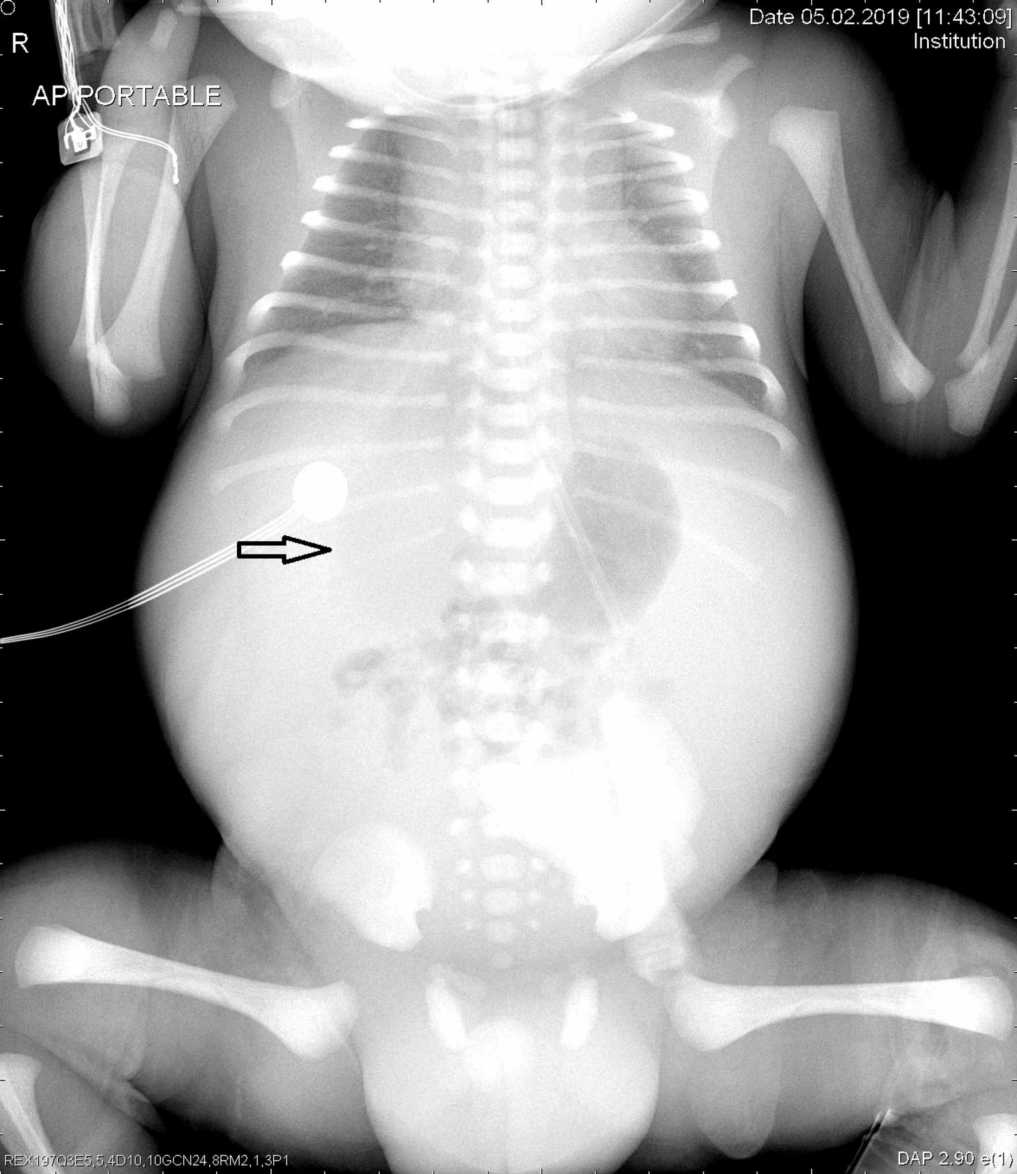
X-ray of abdomen supine. Arrow showing circular faint lucency in the mid abdomen region
